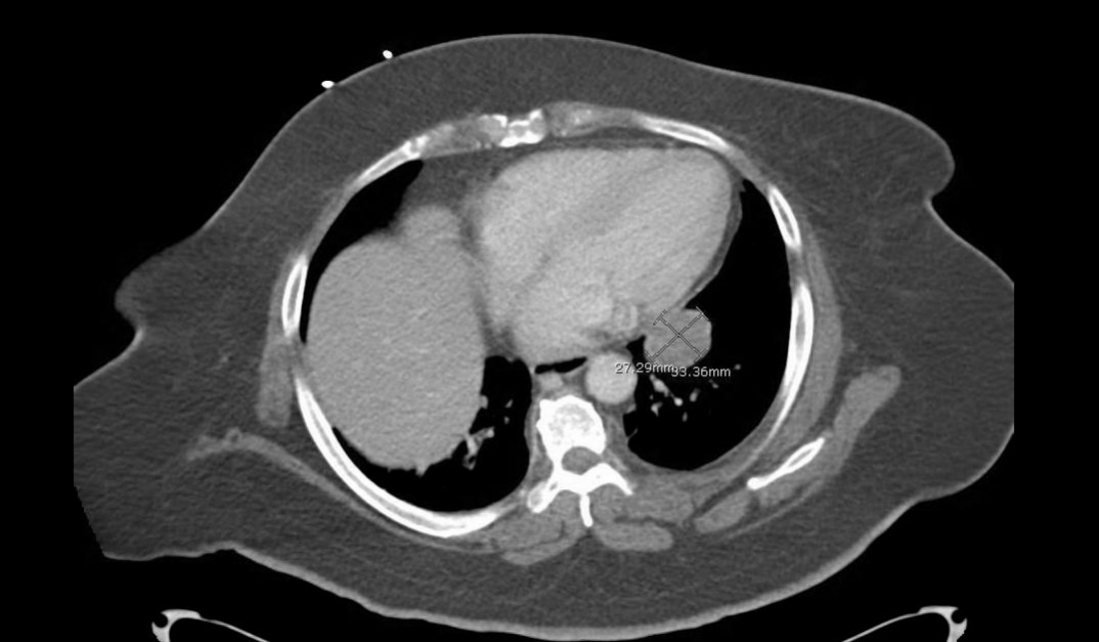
The CT scan of the chest showing a soft tissue attenuating mass in the medial left lower lobe measuring 3.5 x 4.1 x 3.1cm
